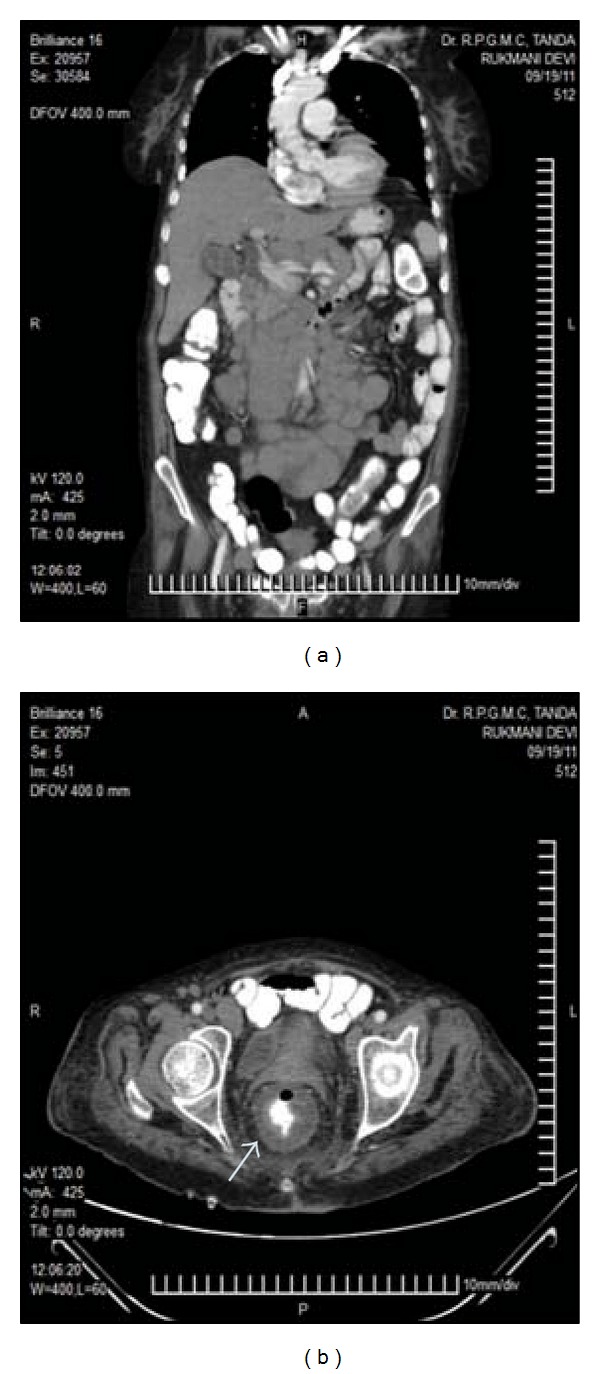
Retroperitoneal lymphadenopathy: multiple enlarged lymph nodes from epigastrium down to aortic bifurcation (a), and thickened sigmoidorectal wall (*arrow*) (b) in CT scan of abdomen.
